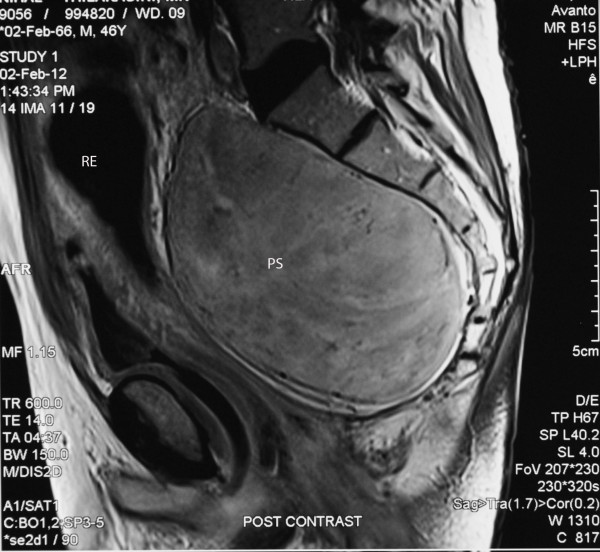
Magnetic resonance imaging of the presacral pelvic schwannoma (PS) compressing the rectum (RE); sagittal section.
